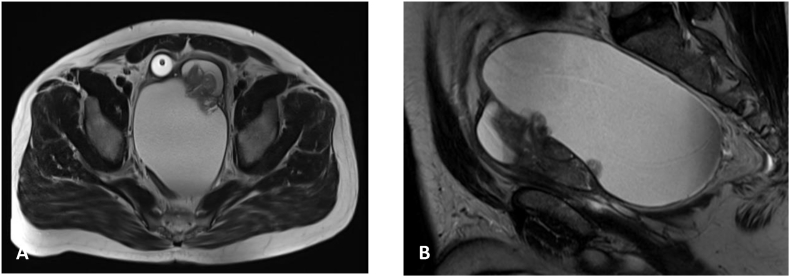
Follow up MRI showed large seminal vesicle cyst on axial (A) and sagittal (B) sections.
